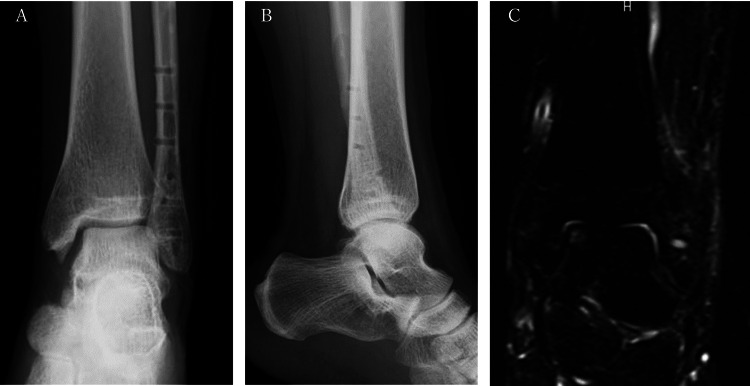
The patient's imaging results at the time of the final follow-up (A) Plain X-ray frontal view; (B) Plain X-ray lateral view; (C) MRI short tau inversion recovery (STIR) image coronal section shows that the bone marrow edema of the talus had almost disappeared.
